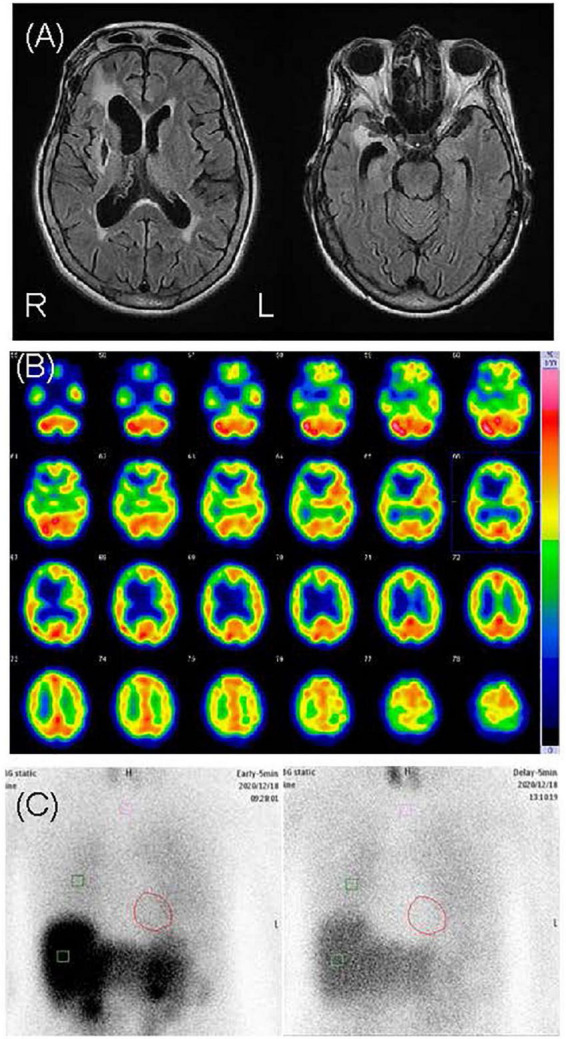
Neuroimages of case 2. (A) Cranial magnetic resonance revealed right dominant bilateral diffuse atrophy and right putamen chronic hemorrhage. (B) N-isopropyl-p-[^123^I] iodoamphetamine single-photon emission computed tomography revealed hypoperfusion in the right basal ganglia and the frontal, temporal, and parietal lobes. (C) Cardiac [^123^I]-metaiodobenzylguanidine scintigraphy revealed heart-to-mediastinum ratios of 1.91 and 1.32 in the early and delayed phases, respectively.
